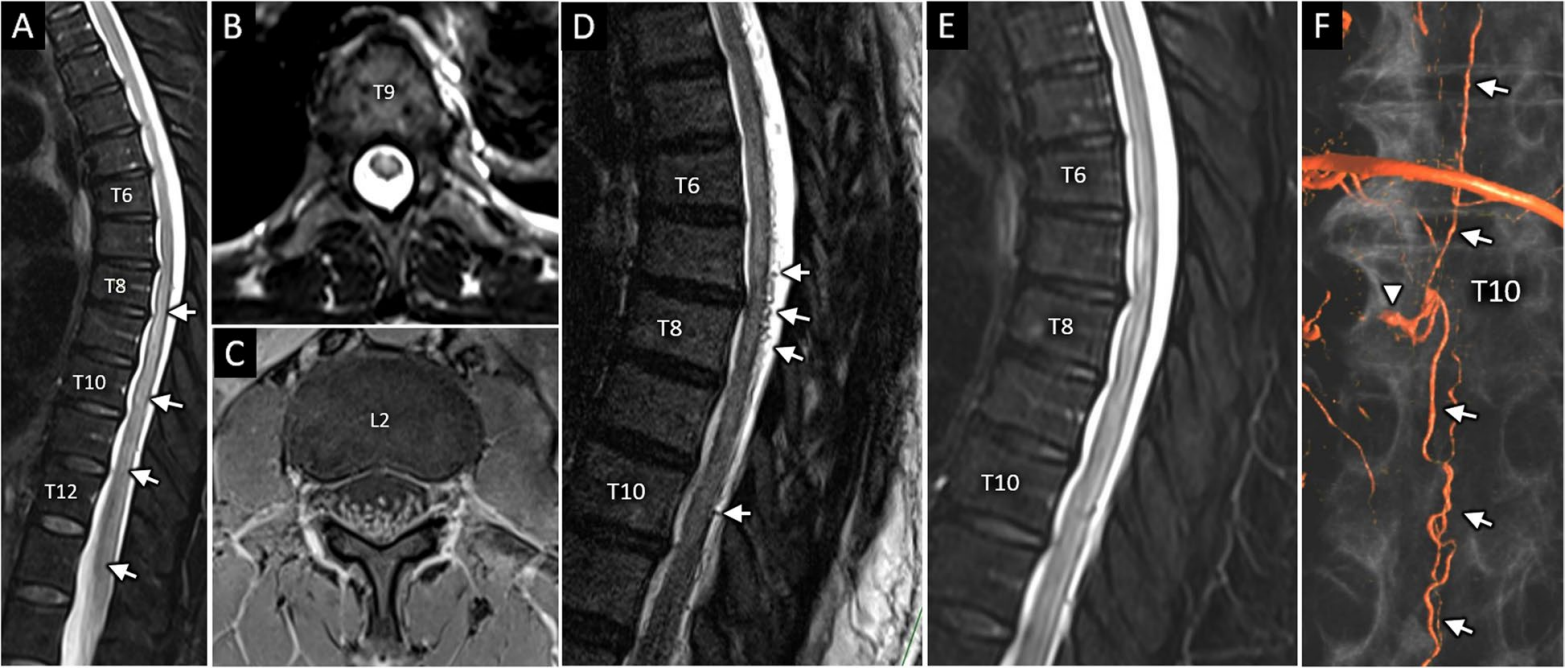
MRI characterization of SDAVF. Sagittal (A) and axial (B) T2-weighted images of the thoracic spine on day 3 reveals longitudinally extensive centromedullary T2 hyperintensity extending from T8 through the conus (A, arrowheads). Axial T1 post contrast image (C) at L2 shows diffuse abnormal thickening and hyperenhancement of the cauda equina nerve roots. Follow-up MRI total spine on day 32, which included high resolution sagittal 3D T2-SPACE sequence (D), clearly depicts numerous dilated vascular flow voids along the dorsal spinal cord surface (D, arrowheads). These abnormal vessels are not well resolved with the conventional T2 sequence (E) acquired as part of the same exam and registered to the same level of the thoracic spine shown in panel D. 3D volume-rendered reformatted image (F) from conventional digital subtraction angiogram reveals right T10 shunting AVF (arrowhead) with multiple dilated pial vessels extending craniocaudally along the spinal canal (F, arrows), confirming diagnosis of SDAVF
